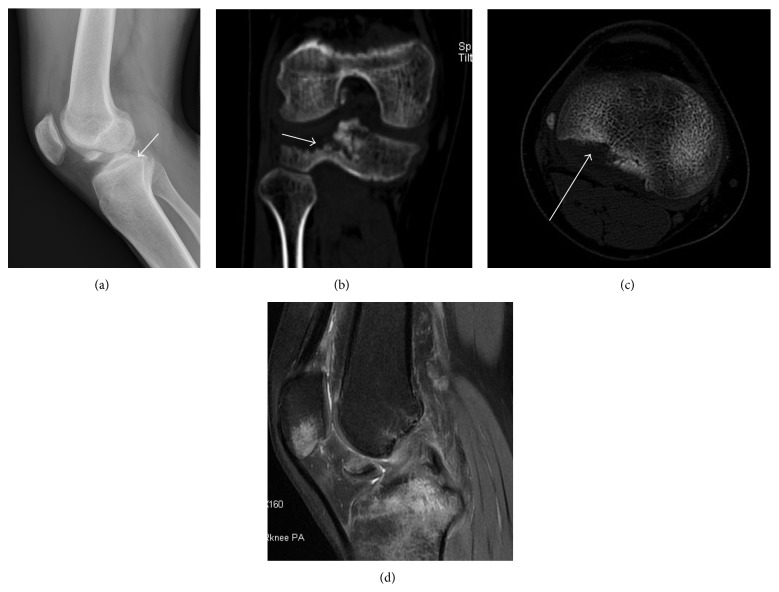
Imaging examinations of the first patient. The X-ray image (a) shows a posterolateral tibial plateau fracture (white arrow). In CT scans (b and c), a posterolateral tibial plateau fracture is found (white arrow). In the MRI image (d), a tortuous ACL was confirmed with the patella and tibia had a bone bruise. No patella fracture was found. There was a fracture fragment in the notch.
